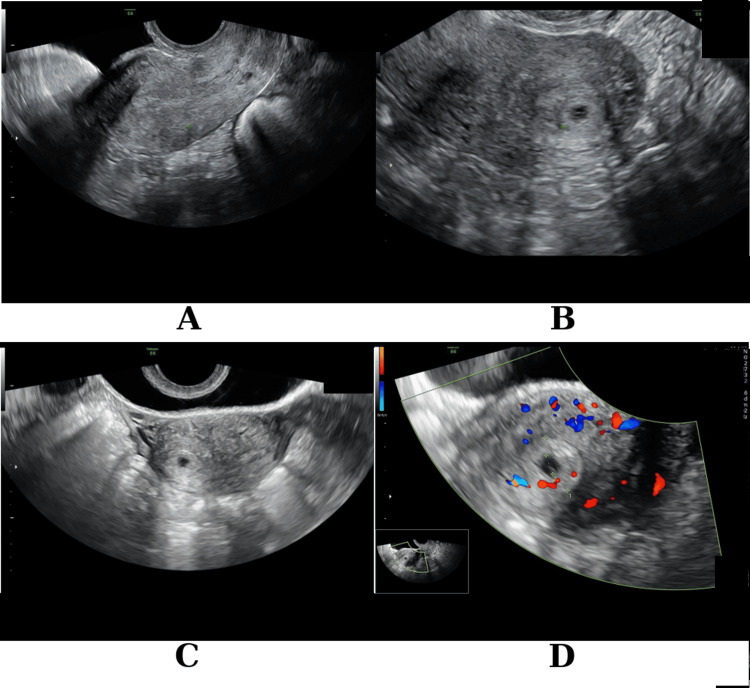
Transvaginal ultrasound findings of angular pregnancy with myometrial extension (A) Empty endometrial cavity; (B) Eccentrically located gestational sac; (C) Apparent intramyometrial localization of the gestational sac, mimicking an interstitial implantation; (D) Color Doppler imaging demonstrating persistent peripheral vascularization of the gestational sac following systemic methotrexate administration.
